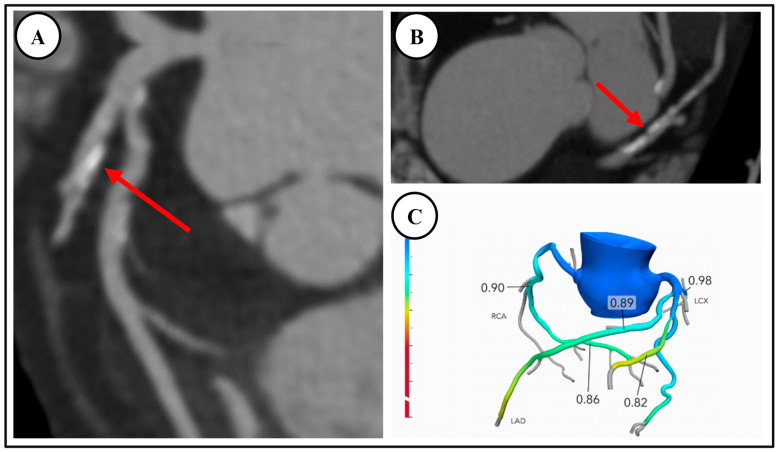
A 75-year-old outpatient with stable angina, with CT demonstrating moderate CAD without ischemia. (A,B) Coronary CT angiography demonstrated moderate coronary artery disease, stenosis 50% with mixed disease in the proximal and mid-left anterior descending artery, red arrows (CAD-RADS = 3). (C) FFR-CT negative for obstructive disease. Patient subsequently underwent stress echocardiography which was negative for inducible ischemia. He was commenced on aggressive risk factor modification.
